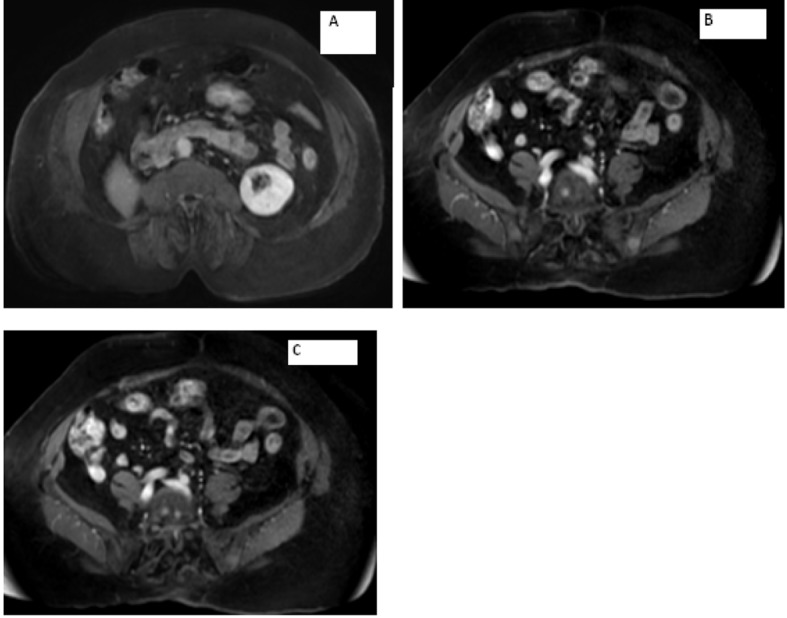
Magnetic resonance imaging of the abdomen showing (A) empty right adrenal and renal fossa and (B, C) metastasis seen in the vertebrae.
